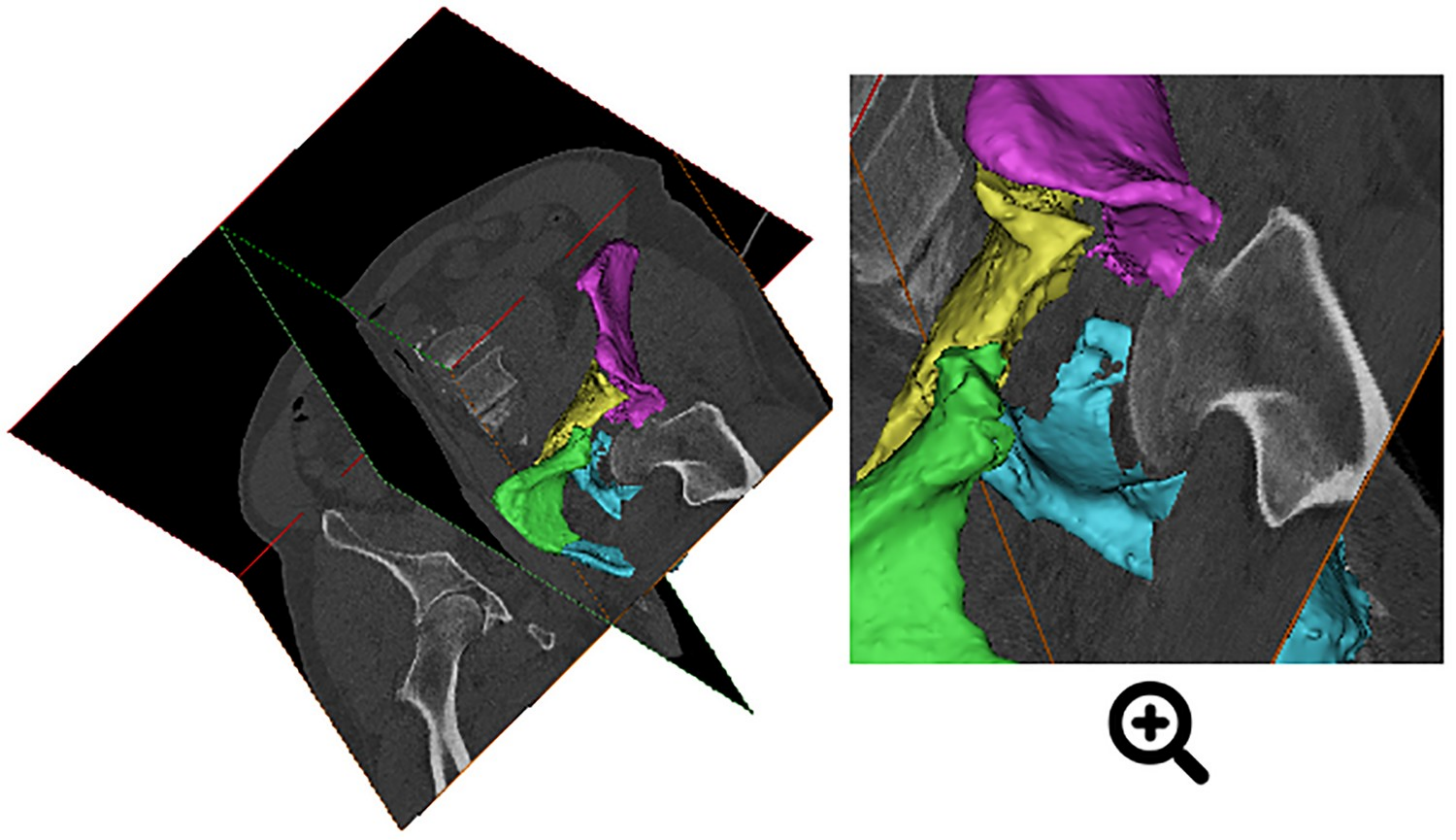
Visualization. Dual projection of a preoperative CT scan of an acetabular fracture with a superimposed 3D model, generated from the CT data.
